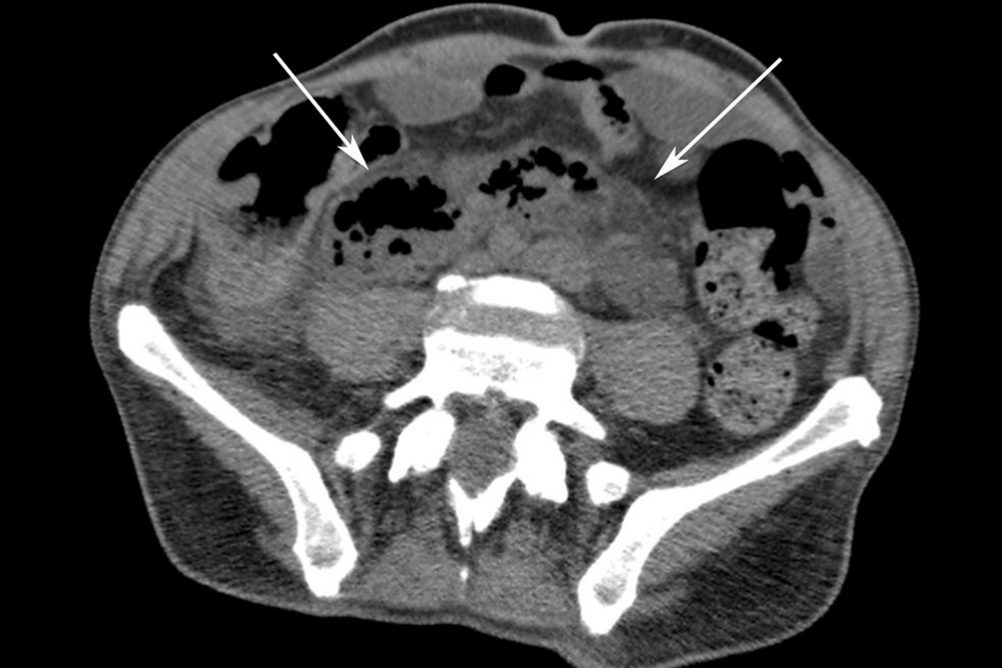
Axial venous phase contrast-enhanced CT image showing a large retroperitoneal abscess containing air pockets (arrows).
